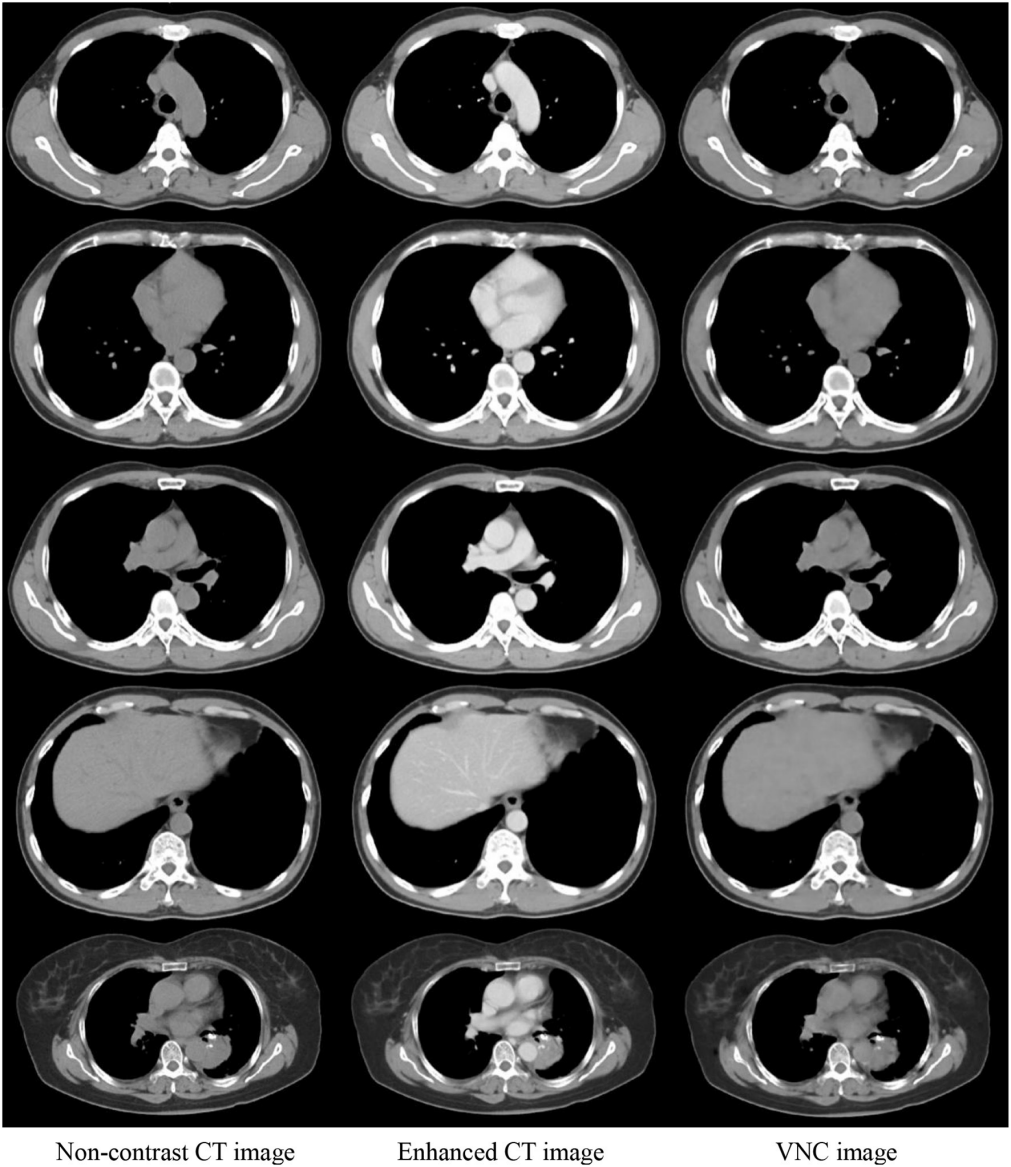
Comparison of three CT images in the same slice. Real non-contrast CT, enhanced CT, and VNC images generated through U-Net at the left, middle, and right columns, respectively.
